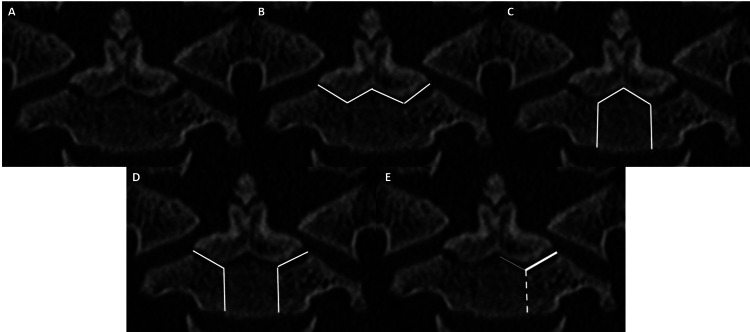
Coronal CT (A) shows calcified os terminale with unfused apicodental synchondrosis. Remaining synchondroses also incompletely fused, as expected for age. Coronal CT image (B) demonstrates a theoretical type 1 fracture through bilateral ondontoneural and midline odontocentral synchondroses (white line). Coronal CT image (C) demonstrates a theoretical type 2F fracture through bilateral neurocentral and midline odontocentral synchondroses (white line). Coronal CT image (D) demonstrates theoretical type 3 fracture through bilateral neurocentral and odontoneural synchondroses (white lines). Coronal CT image (E) demonstrates a theoretical type 4 fracture through either odontoneural synchondrosis with or without adjacent injury to bordering odontocentral or neurocentral synchondrosis. Provided image depicts left odontoneural (thick, solid line) and possible injury to adjacent odontocentral (thin, solid line) or neurocentral (dashed line) synchondroses. CT - computed tomography
